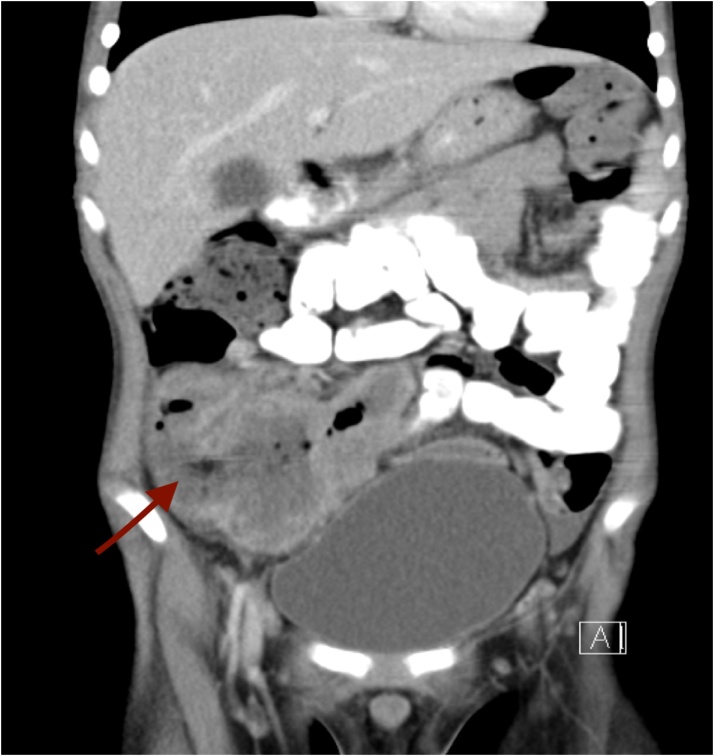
CT scan showing a large complex lesion in the right iliac fossa displacing the bladder and bowel loops medially.
